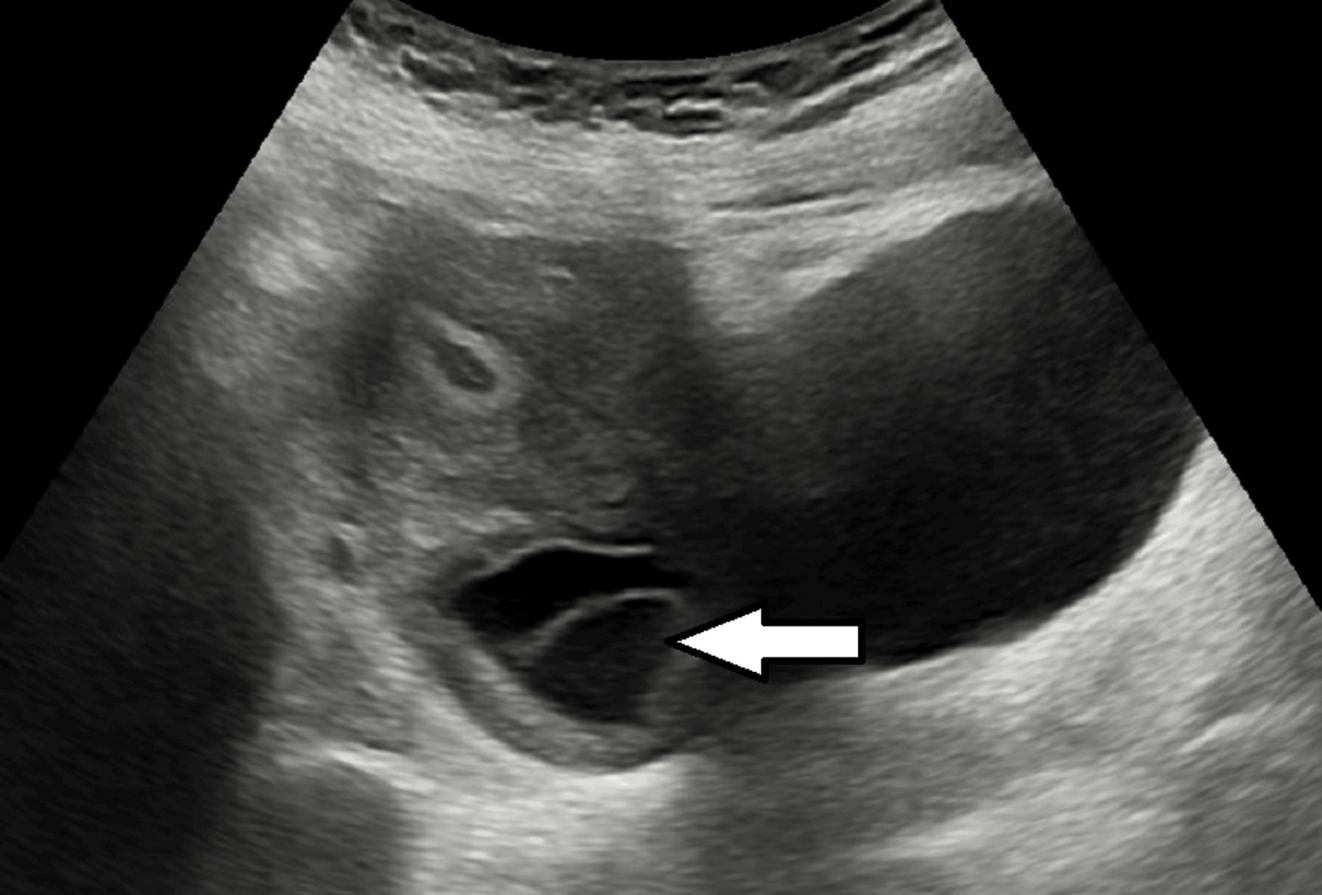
Ultrasound of the cervix showing a multiseptated cystic lesion.
